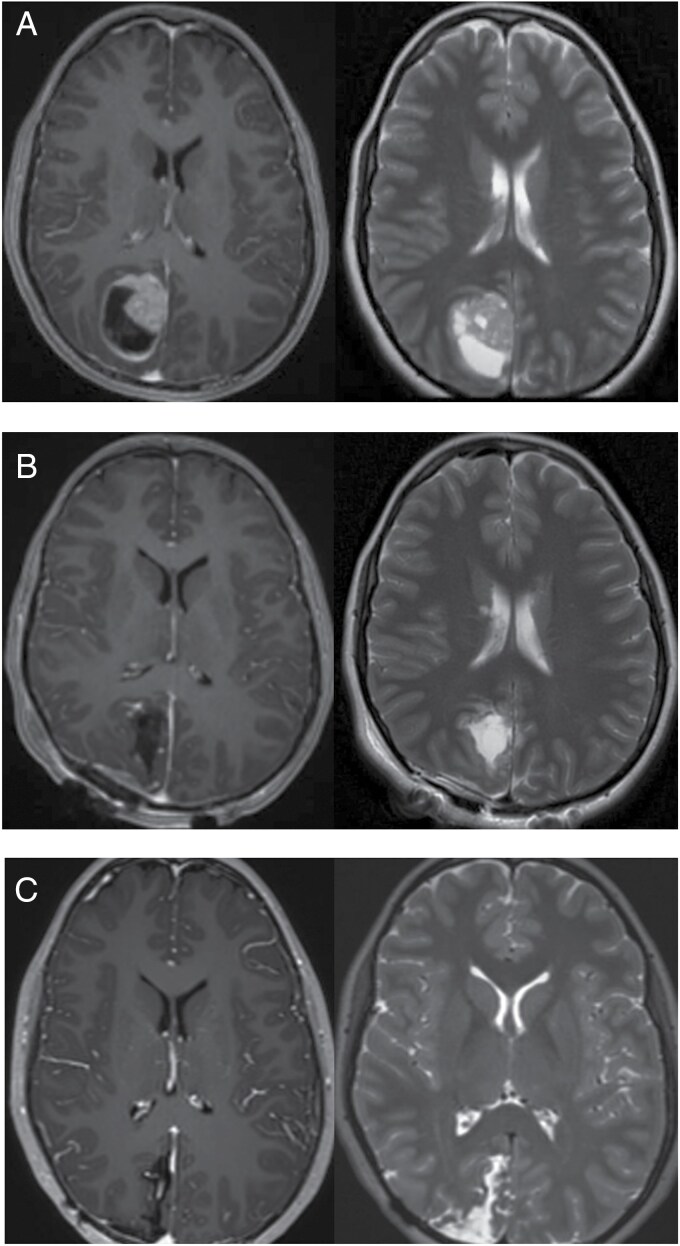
(A) Initial T1 enhanced (left) and T2 (right) MRI brain imaging revealing circumscribed right occipital lobe mass. (B) Post-resection T1 enhanced (left) and T2 (right) MRI brain imaging. (C) Most recent surveillance T1 enhanced (left) and T2 (right) MRI imaging.
